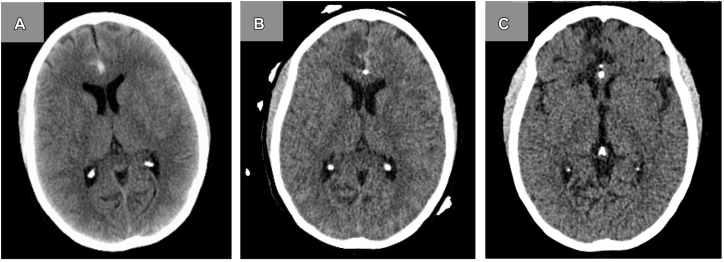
Serial noncontrast CT axial head images. A, Initial presentation with diffuse subarachnoid hemorrhage within an interhemispheric fissure. B, One day postoperative following stent and coil embolization of 2 right A2-3 junction aneurysms. Decreased density is consistent with the evolution of clot. C, Eight-week follow-up showing chronic infarction of ACA territory. *ACA*, Anterior cerebral artery; *CT*, computed tomography.
